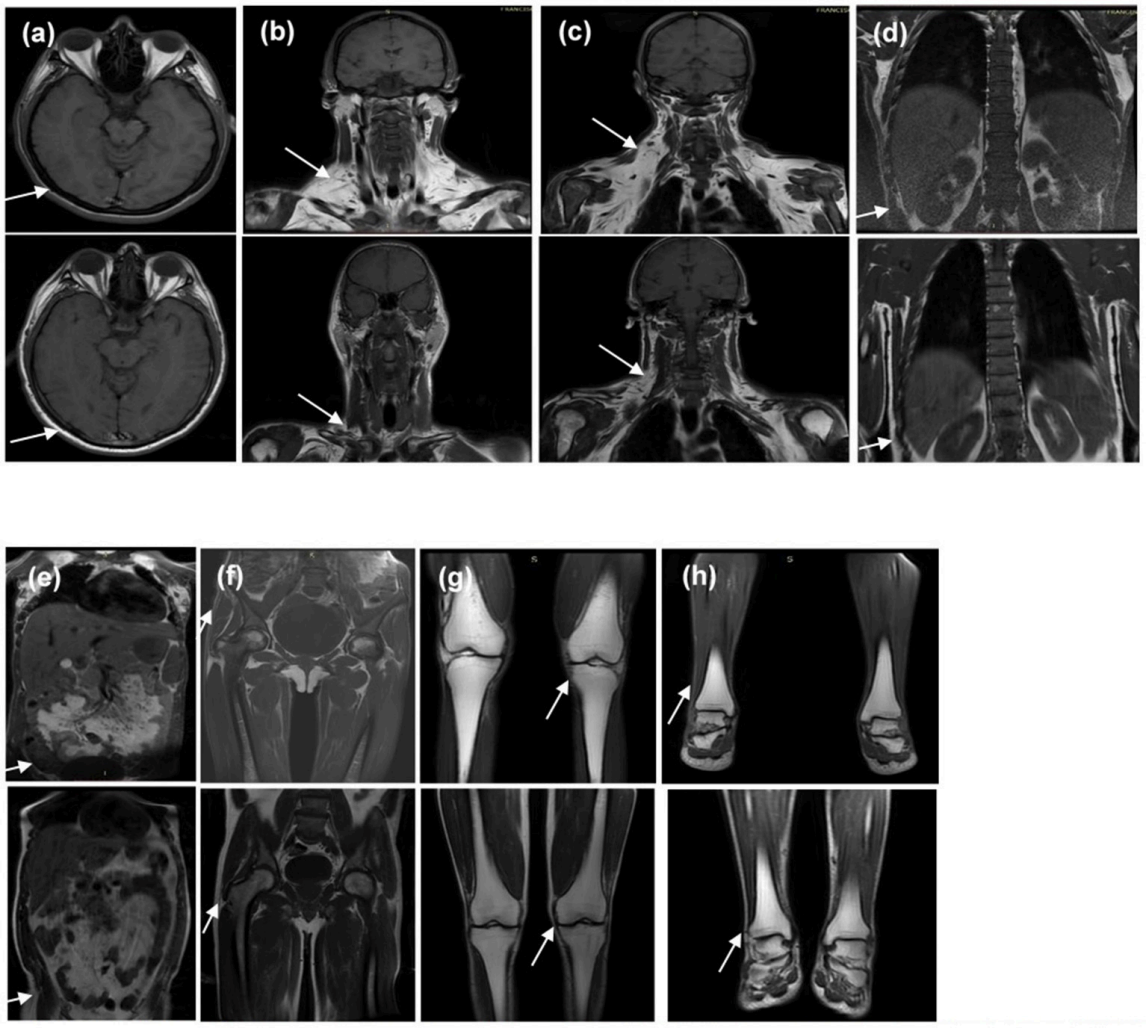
T1-weighted magnetic resonance imaging of the (1) homozygous proband's sister and (2) the heterozygous father at the levels of (a) orbits and scalp, (b) anterior neck, (c) dorsocervical, (d) thoracolumbar spine, (e) abdomen, (f) pelvis and thighs, (g) knees, and (h) feet. It is worth noting that the sister and father's body mass indices are 20.7 and 24.9 kg/m^2^, respectively. Note the diffuse absence in subcutaneous tissue of the homozygous proband's sister (indicated by the arrows in a,d–h) and excess fat deposition in the neck and dorsocervical area (indicated by the arrows in b,c).
